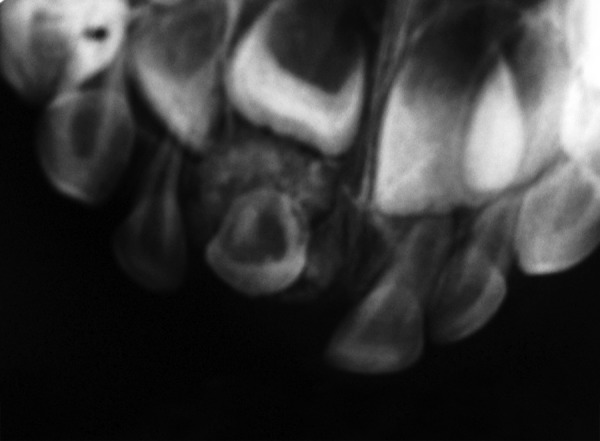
Preoperative X-ray showing the unerupted primary incisor and the surrounding bone
